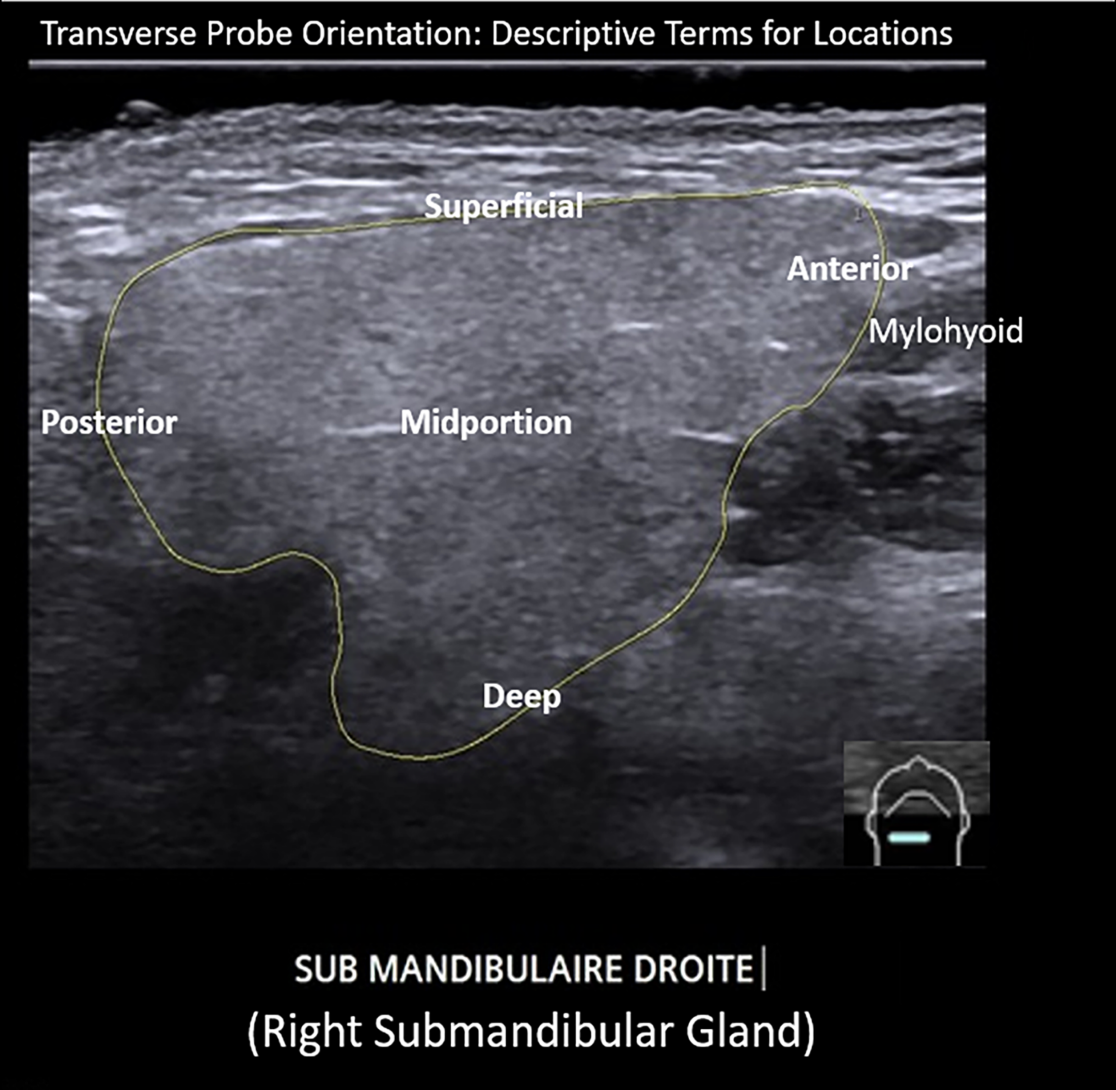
Ultrasound image employing transverse orientation of the probe identifies regions within the outlined right submandibular gland that are labeled with descriptive terms (image courtesy of Dr. Philippe Katz; relabeled).
